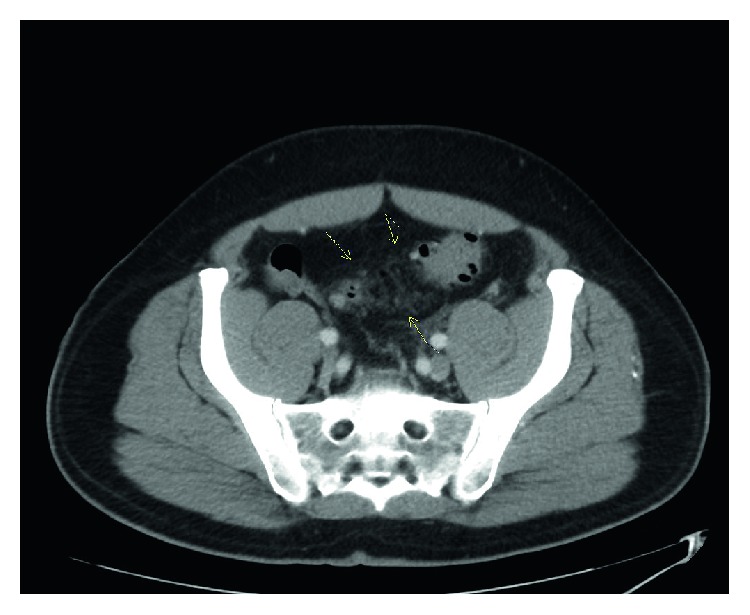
CT abdomen showing pericolonic induration (marked with arrows).
